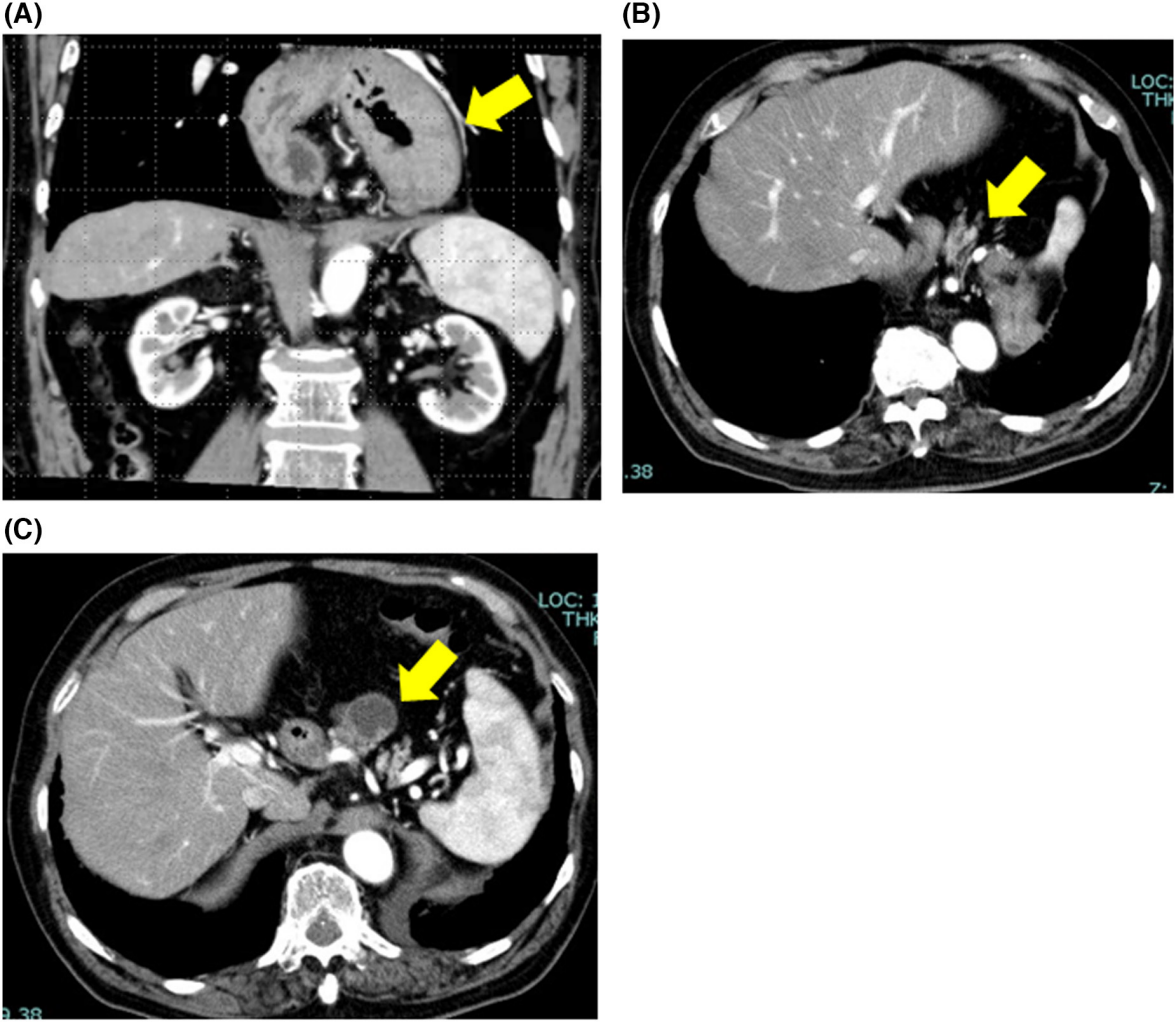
Computed tomography images showing (A) prolapse of the stomach, (B) prolapse of the pancreatic body, and (C) an 8‐mm solid component in the pancreatic body
